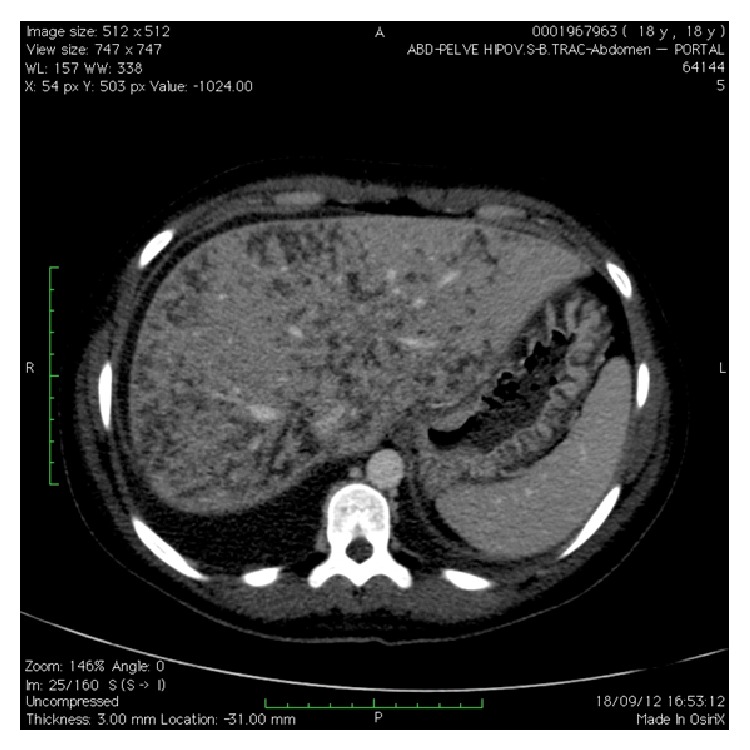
Magnetic resonance imaging on the upper abdomen showing liver with slightly increased dimensions and a regular outline, presenting multiple irregular hypodense areas of serpiginous type that affect the entire liver, without highlighting from the contrast, thus corresponding to areas of hepatic infarction.
